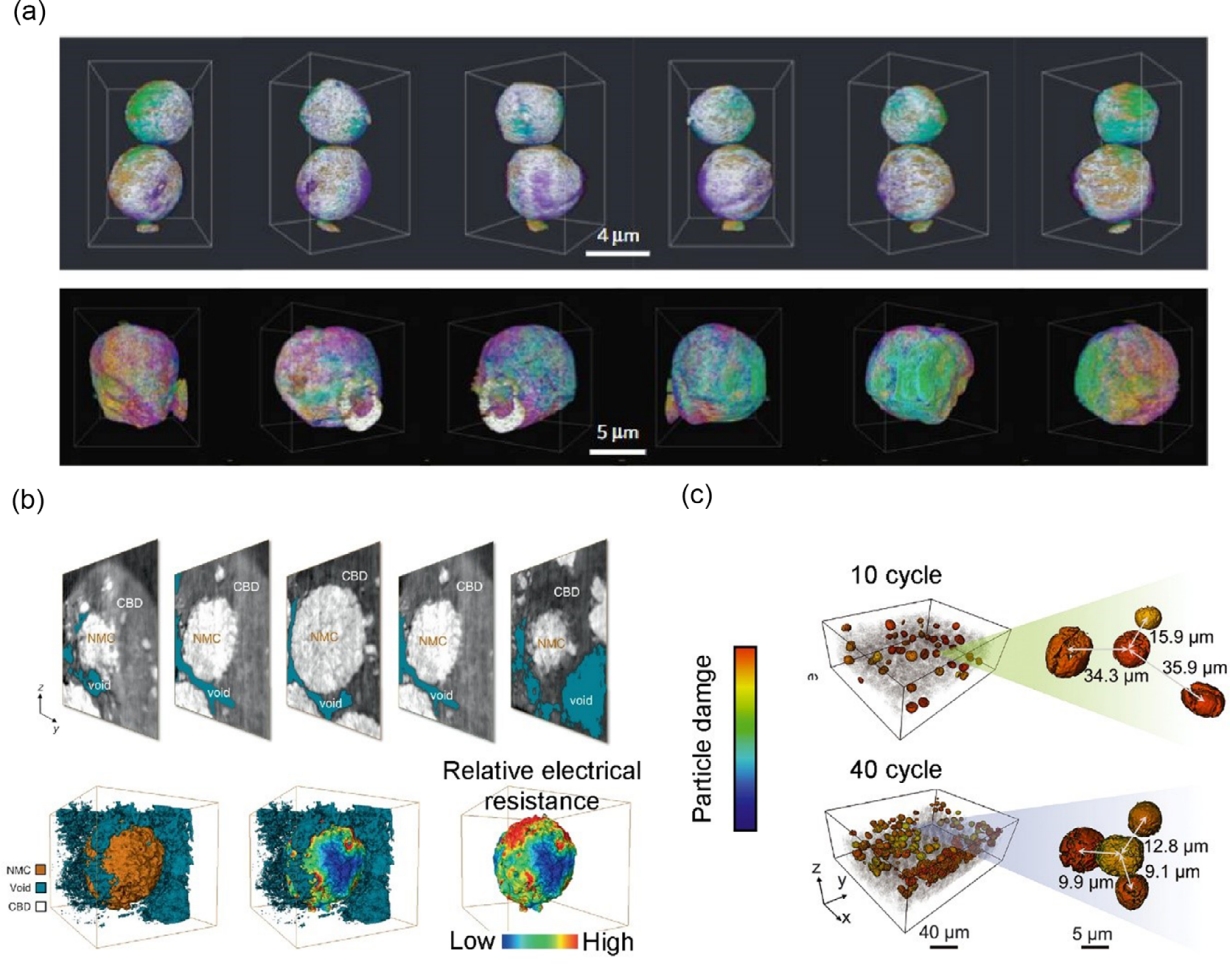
a) 3D rendering of the elemental association maps of NMC particle generated using X‐ray CT. Many nanodomains showed deviation in as‐made precursor and annealed NMC particles. Reproduced with permission.^[^
